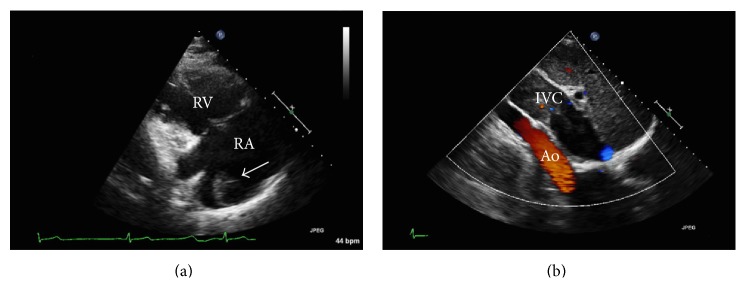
(a) Transthoracic echo showing right atrial mass (arrow). (b) Doppler flow showing IVC tumor restricting blood flow.
